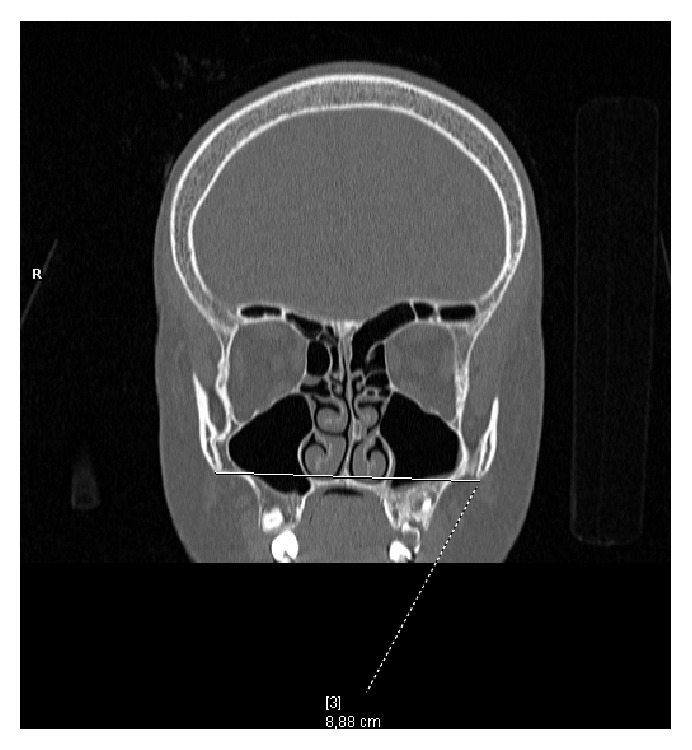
An example of zm/zm distance marked on the CT image.
